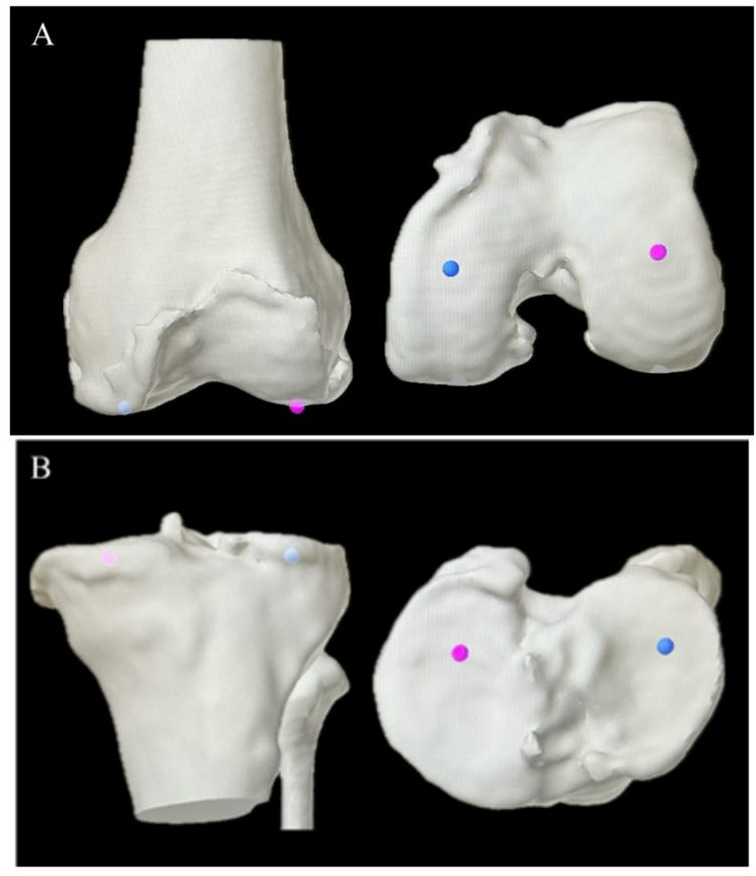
Coronal alignment landmarks on three-dimensional computed tomography reconstructions within the Mako Total Knee application protocol (Mako TKA 1.0 software; Stryker). A Distal femoral landmarks defined at the most distal surface of the medial and lateral femoral condyles. B Proximal tibial landmarks defined at the 66% point, located two-thirds of the distance from the anterior to the posterior cortex on the medial and lateral tibial plateaus
